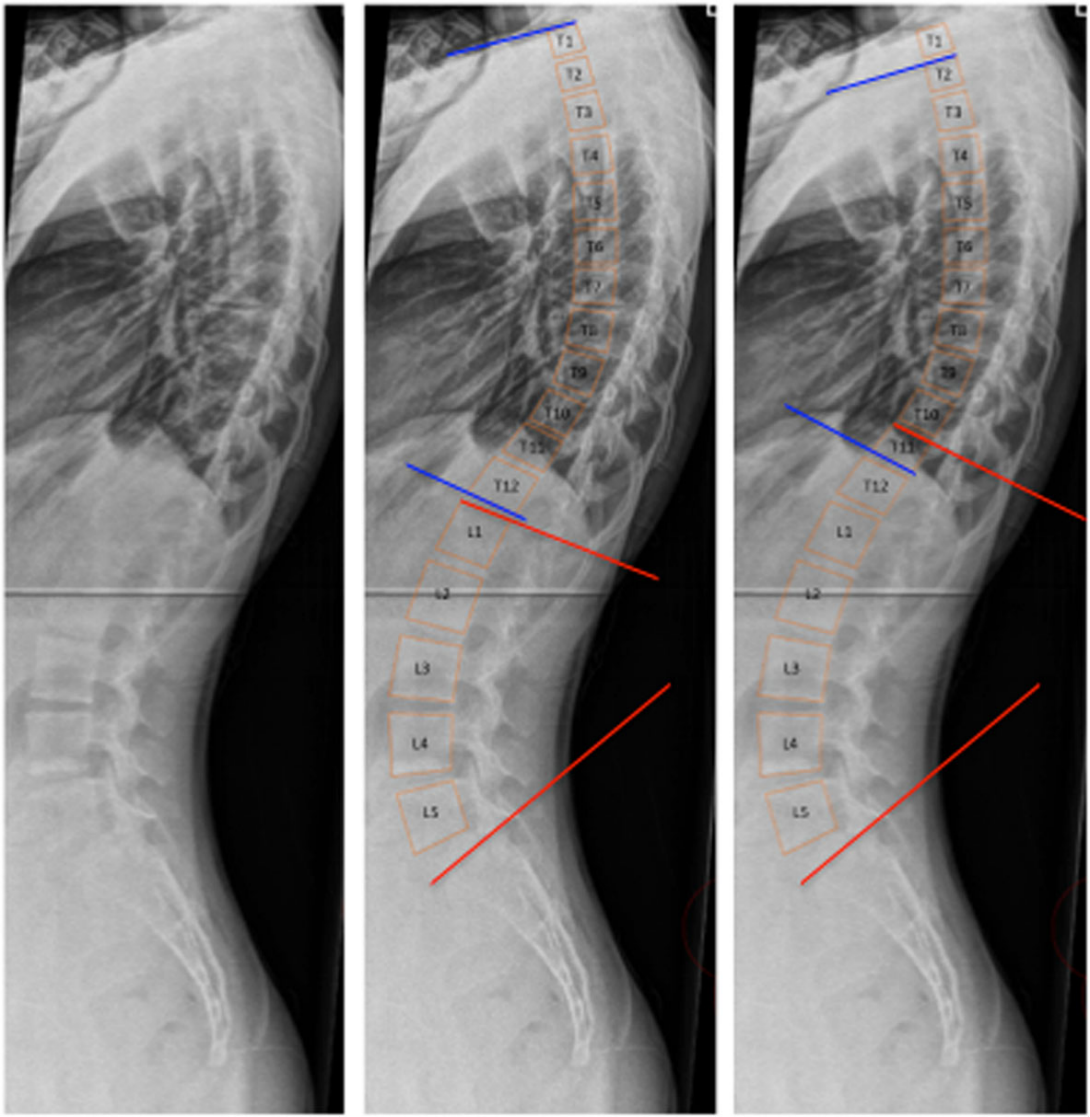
Left*: Standing sagittal radiograph of a thoracic single curve with apex at T8. *Middle*: Fixed measurements of T1–T12 thoracic kyphosis (*blue*) and L1-S1 (red). *Right*: Nonfixed measurements of thoracic kyphosis (*blue*) and lumbar lordosis (*red*)
